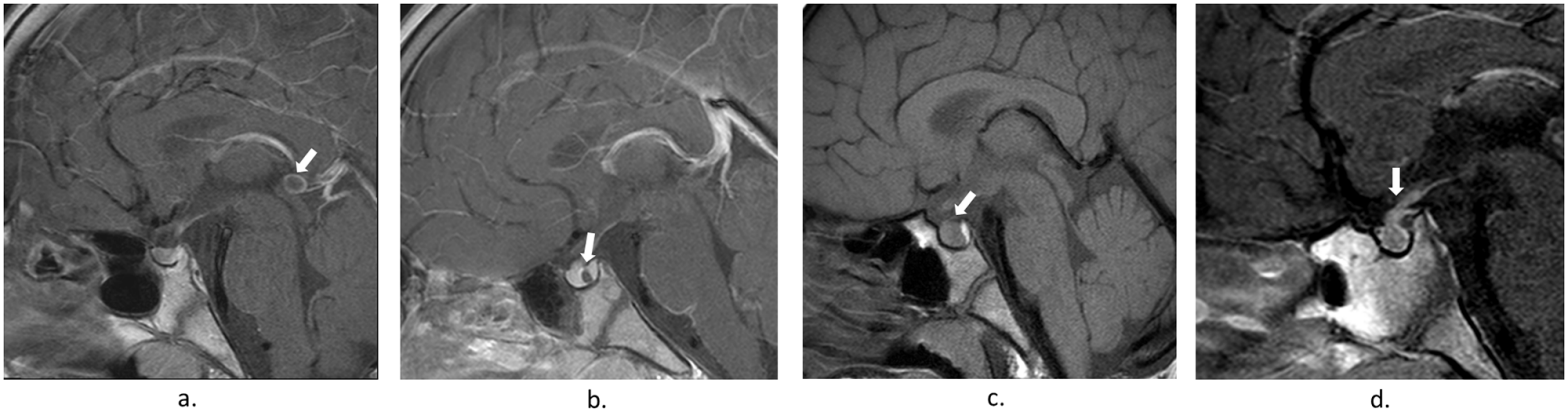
Sellar magnetic resonance imaging of boys with incidental findings. a. Pineal cyst, b. Rathke’s cleft cyst, c. Pituitary hyperplasia, d. Pituitary stalk thickening.
